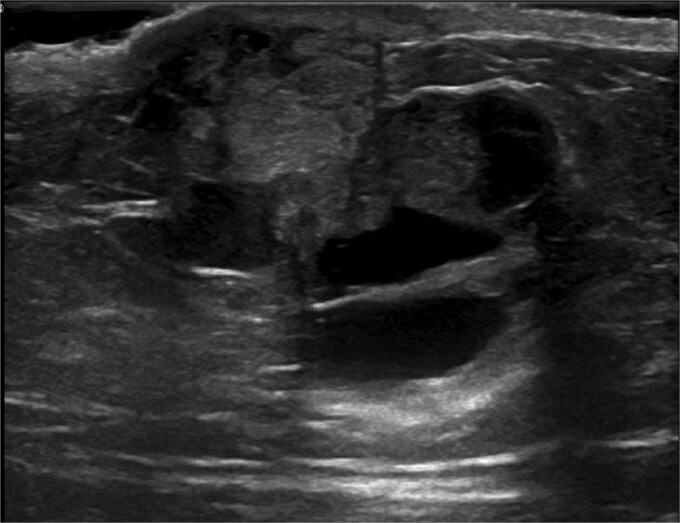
Ultrasound of retroareolar area of palpable concern – first view.
